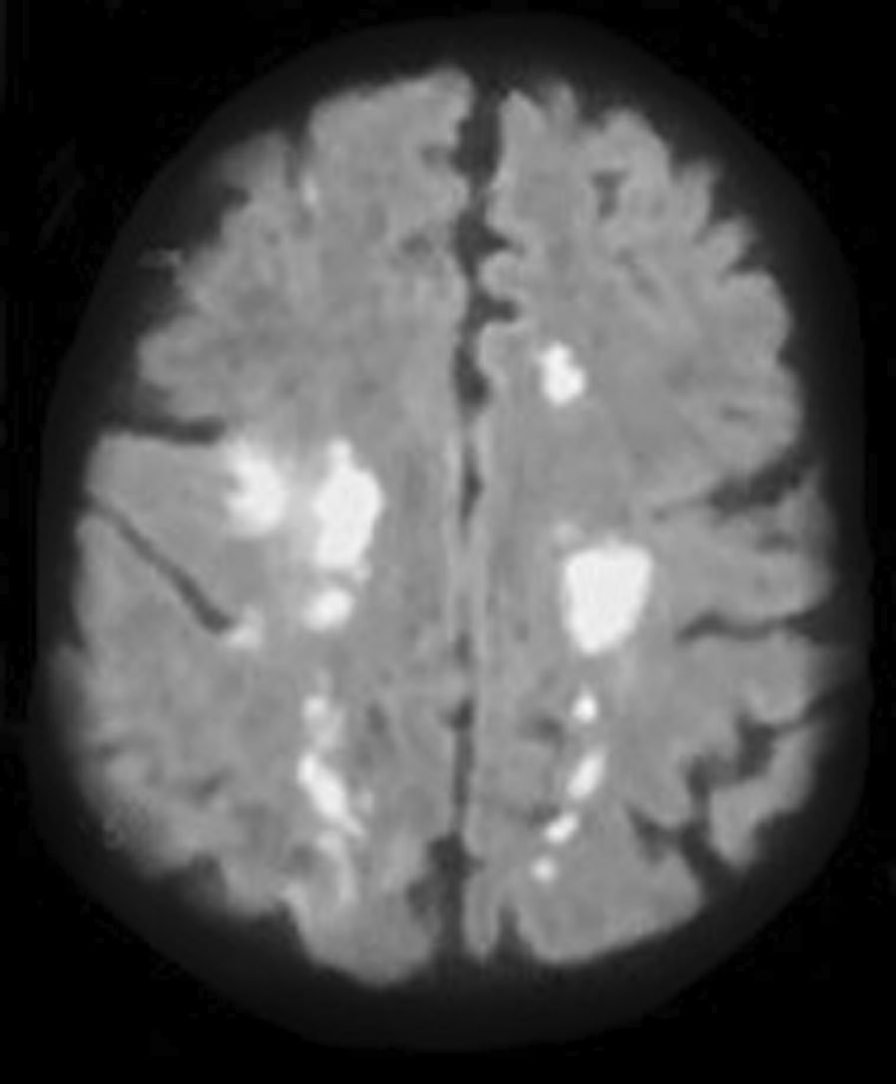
Bilateral cortical border zone (watershed) infarcts as evident by restricted diffusion (DWI)
